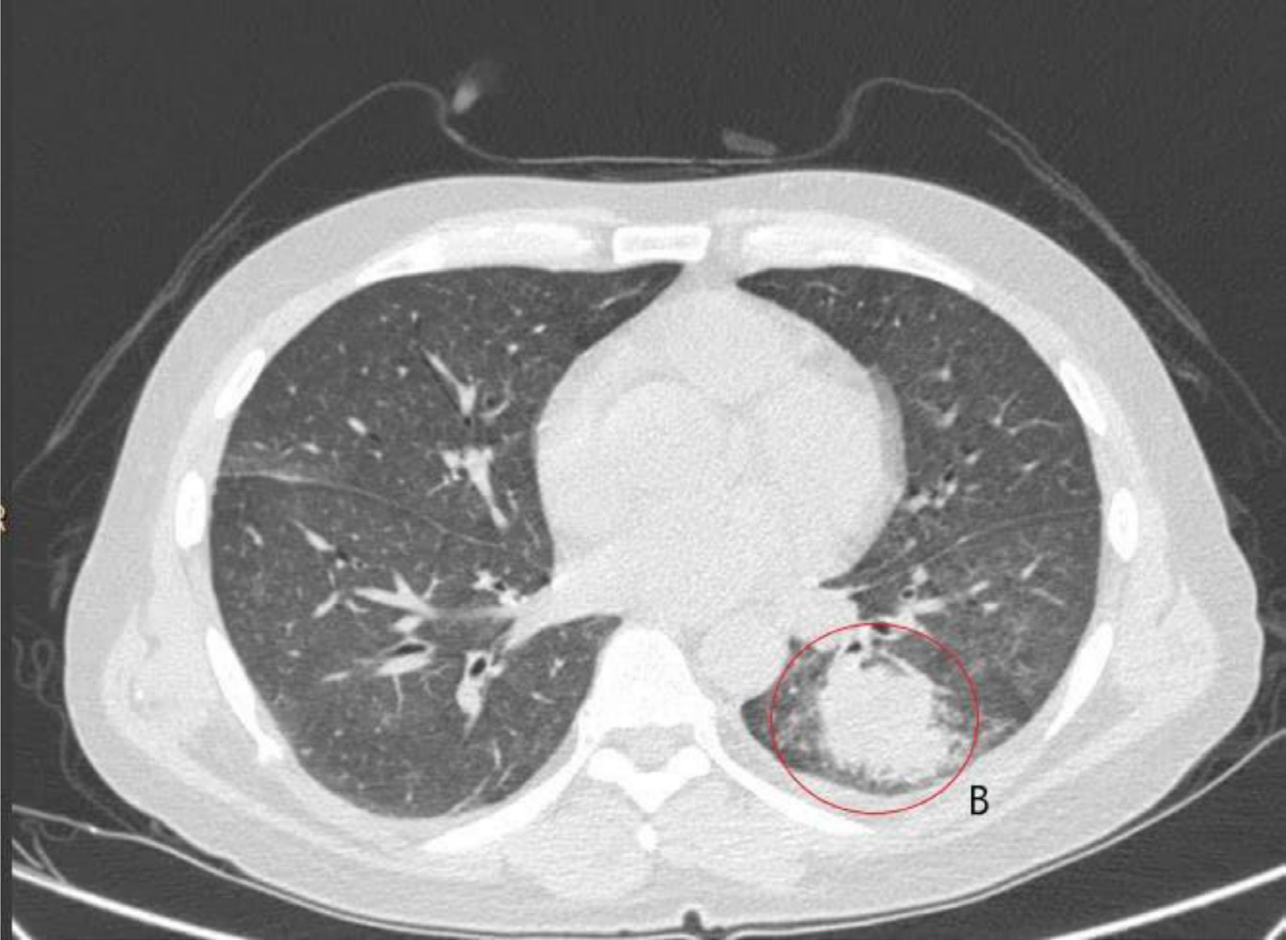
Axial section of computed tomography scan. B encircles the largest consolidation in the left lung.
